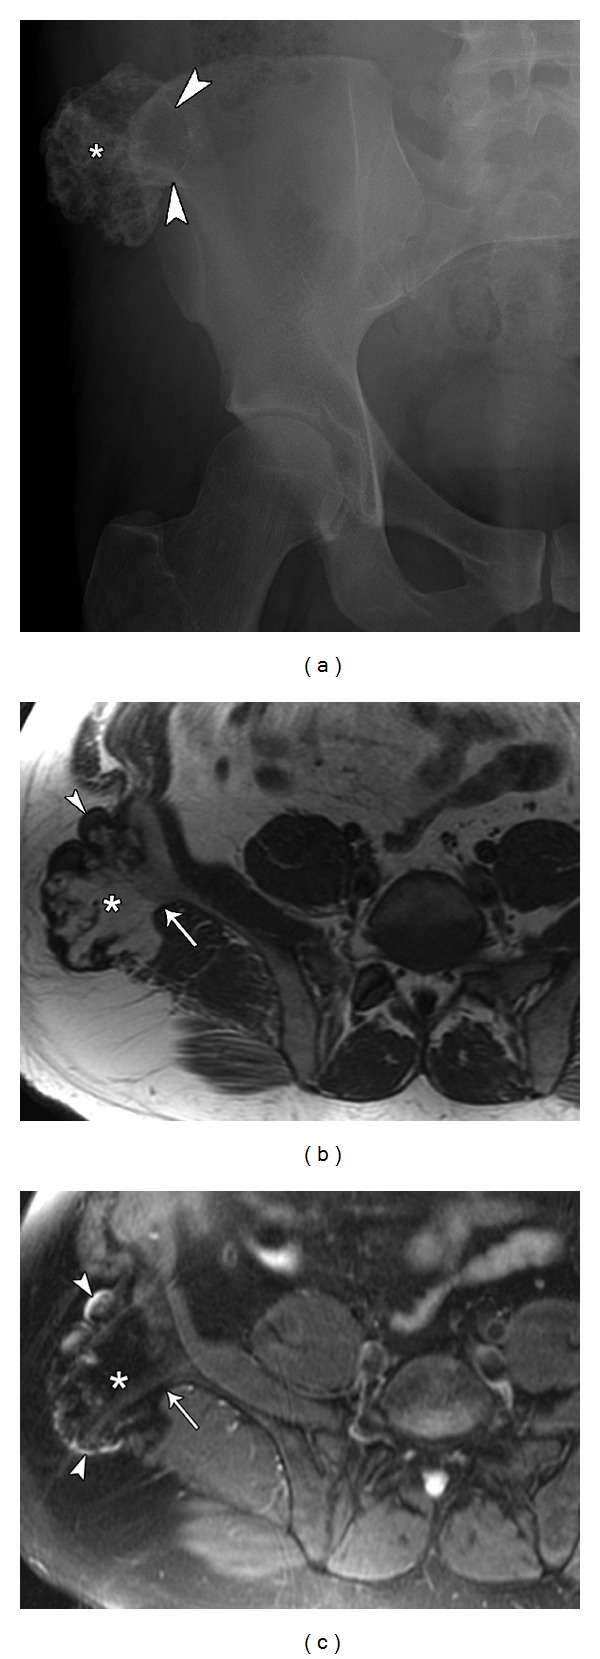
31-year-old male presents with palpable hard mass lateral to right iliac crest diagnosed as an osteochondroma. Pelvic plain film (a) demonstrates an irregularly calcified, pedunculated lesion (asterisk) arising from the right iliac crest. It presents as a peripheral outgrowth with its cortex in continuity with the iliac bone (arrowheads). Axial T1 (b) and axial T2 fat sat. (c) MRI images of same lesion (asterisk) demonstrate the continuity of the cortex and medullary portion of the lesion with the parent bone (arrow) and identify a thin cartilage cap (arrowheads).
